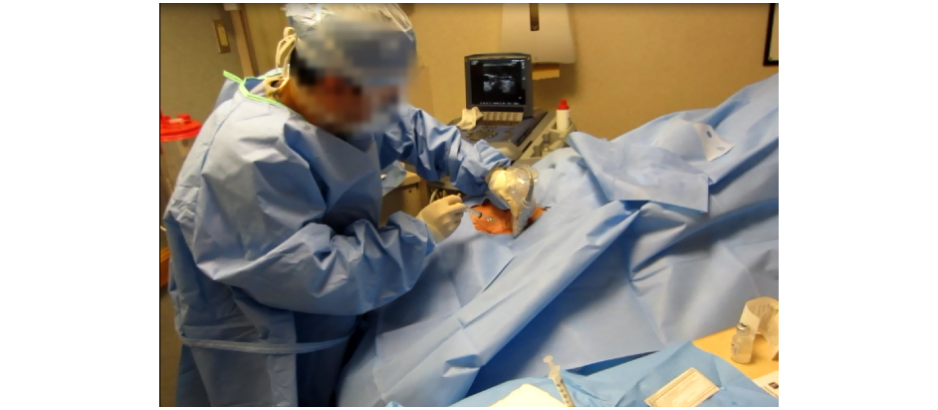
Access needle angle relative to the ultrasound probe.
